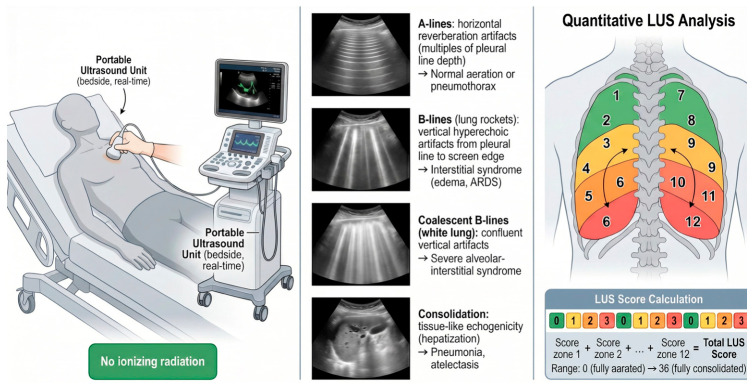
Instrumental setting (Left), artifact classification (Center), and quantitative aeration score (Right) for Lung Ultrasound (LUS). (Left): instrumental setting and patient positioning during LUS examination; (Center): LUS images according to progressive loss of aeration from the top (A-lines) to the bottom (Consolidation). (Right): distribution of lung segment for quantitative analysis of aeration during LUS.
